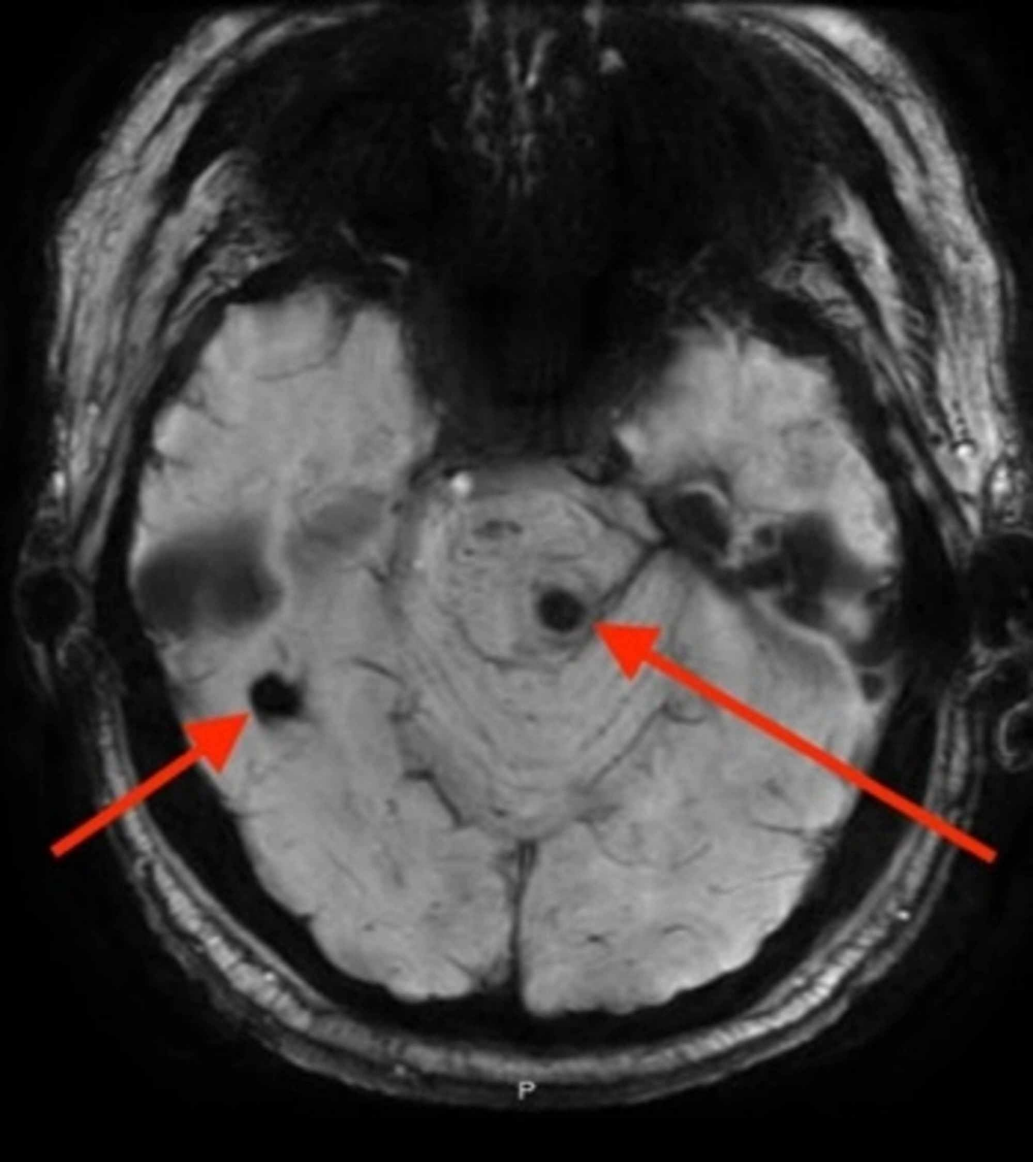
MRI SWI sequence showing multiple areas of hemosiderin deposition consistent with multiple CCMs. SWI: Susceptibility-Weighted Imaging; CCMs: Cerebral Cavernous Malformations.
